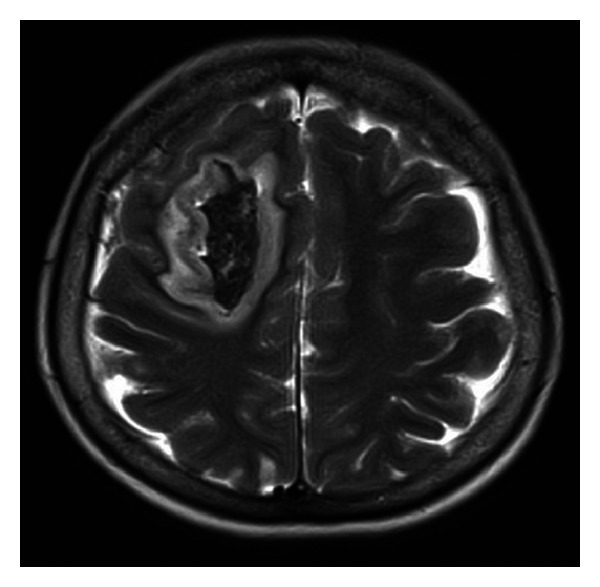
Brain MRI was performed with evidence of intracerebral hematoma with right frontal brain edema.
